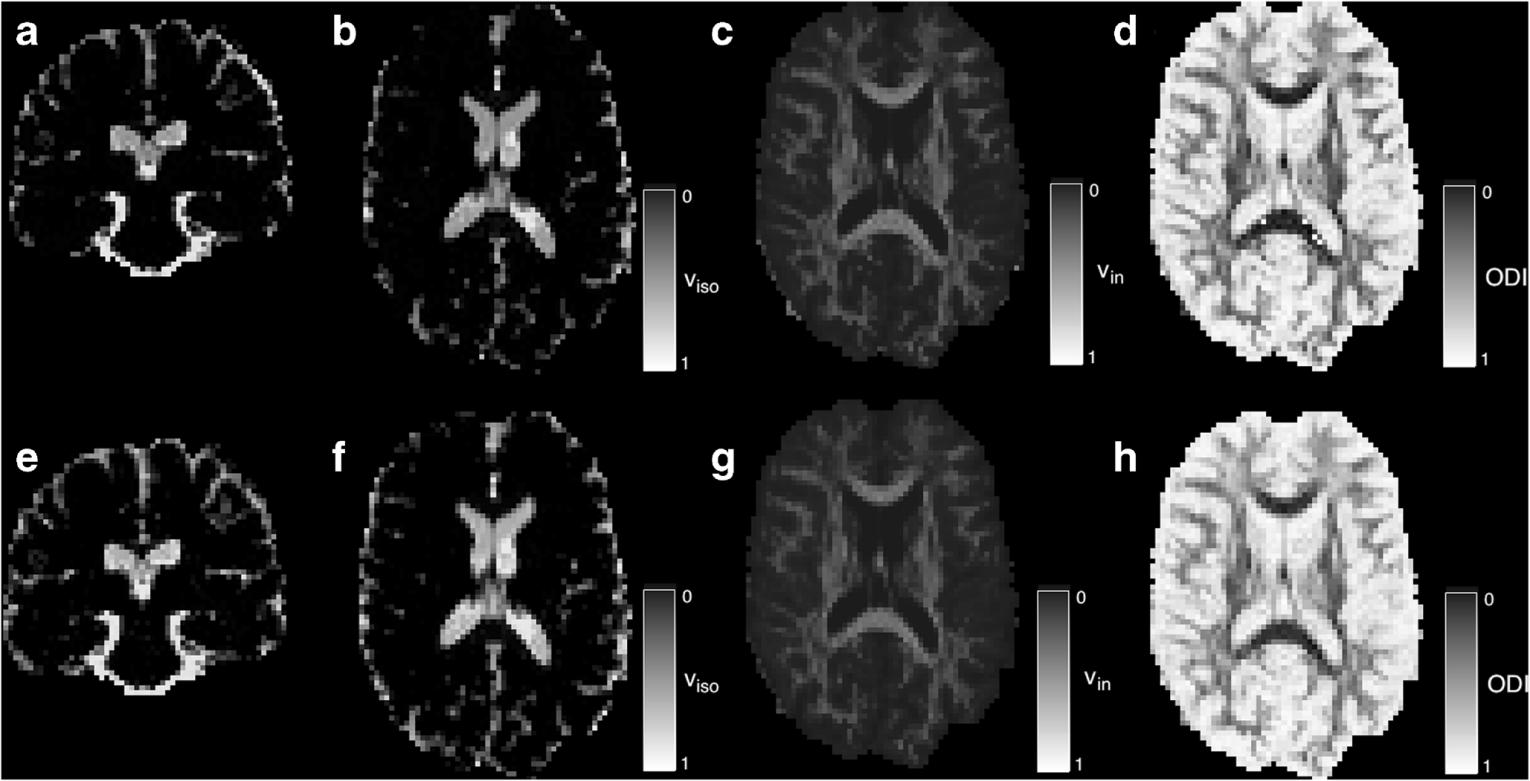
DWI NODDI parameter map comparison between standard and extended fitting. For isotropic volume fraction v_iso_, intra-axonal volume fraction v_in_ and the estimated tissue orientation dispersion index, ODI. Differences can be observed between regions of high *v*
